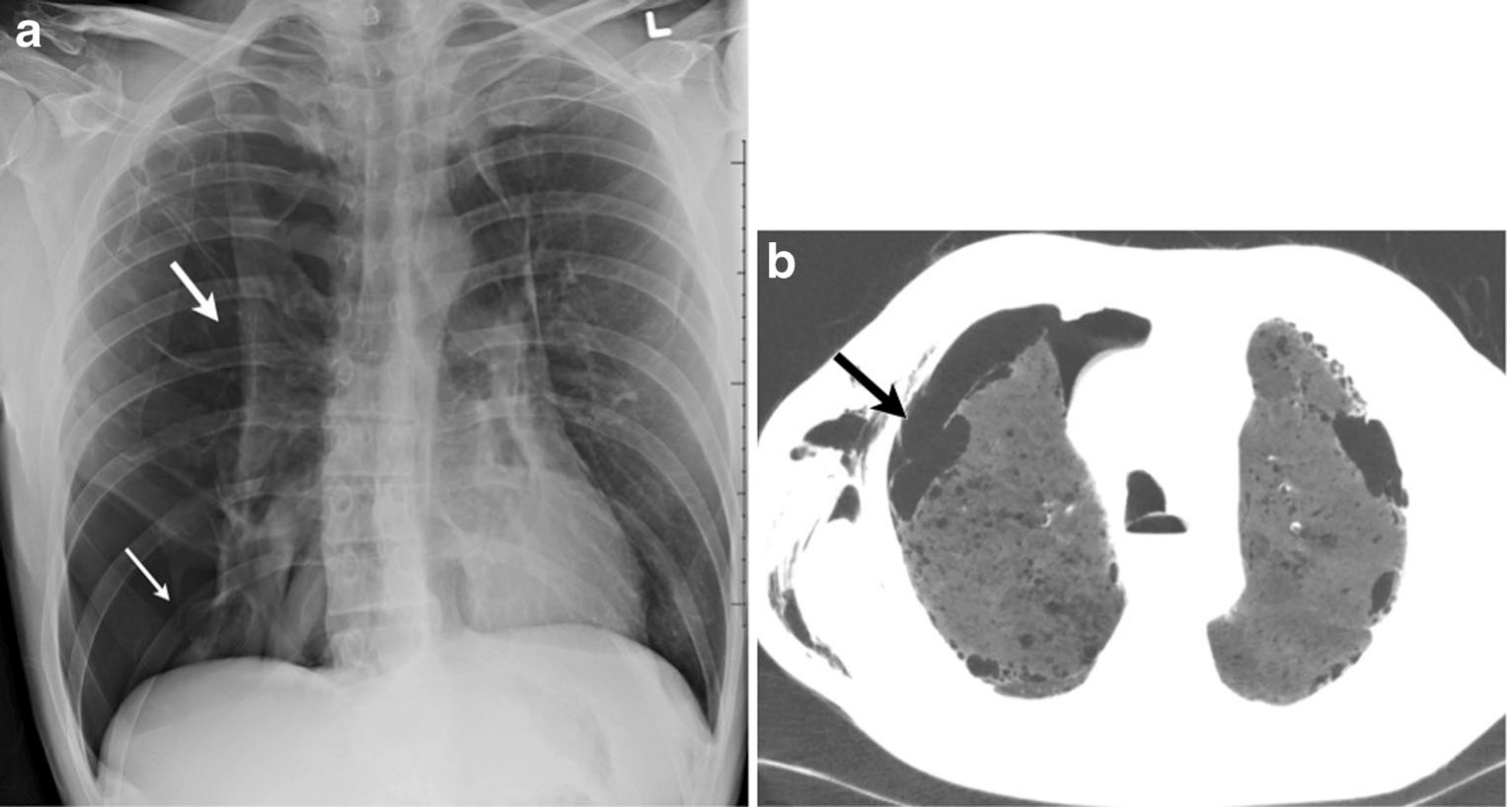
An 83-year-old smoker with chest pain and recurrent pneumothorax. Frontal chest radiograph on admission (a) demonstrates a large right pneumothorax (pleural interface marked by *white arrows*). Axial chest CT minimum intensity projection (minIP) image (b) demonstrates discontinuity of the walls of a bulla (*solid black arrow*) compatible with a ruptured bulla
